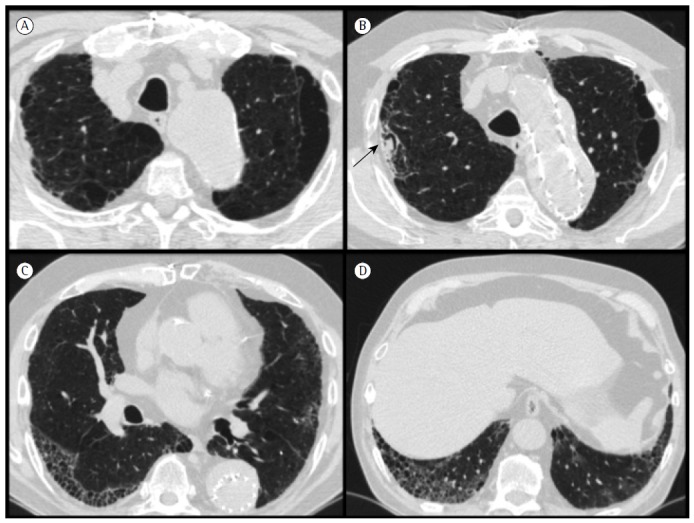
CT scan of the chest of a 67-year-old female patient with combined
